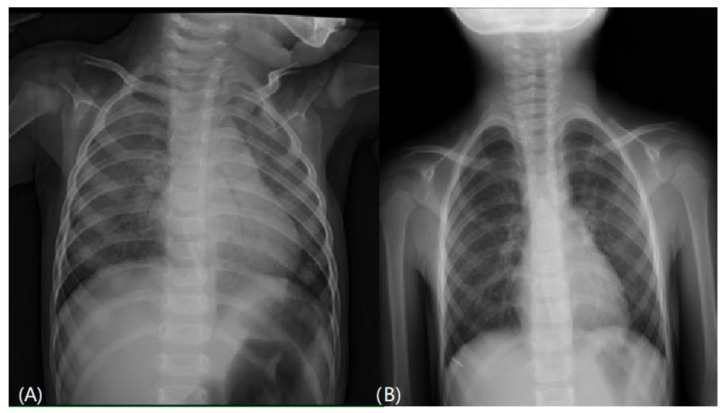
(A) Chest x-ray on admission showed diffuse increased interstitial density of both lungs. (B) Chest x-ray after treatment revealed no radiologic evidence of active cardiopulmonary disease.
